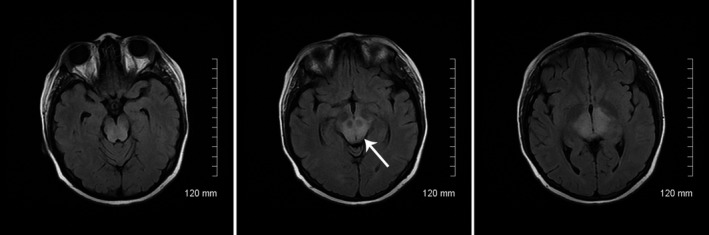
MRI on day 3. MRI T2‐weighted‐FLAIR (fluid‐attenuated inversion recovery) showed midbrain hyperintensity sparing the red nuclei which are hypointense (image in the center)
